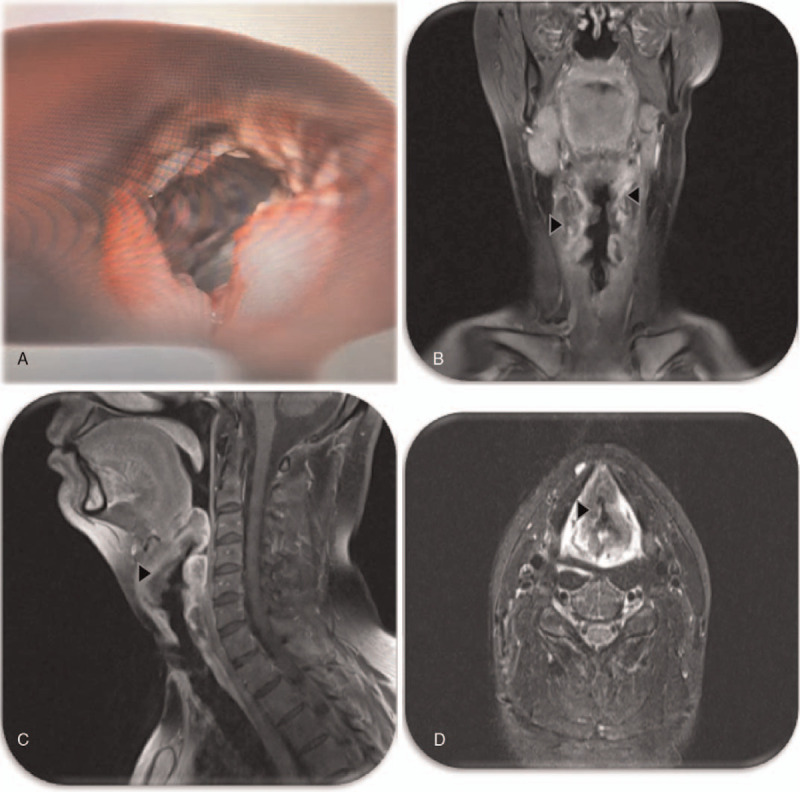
(A) Direct laryngoscopy examination with ulcerative and necrotic lesions at the epiglottis and larynx and loss of the larynx's structure. (B-D) MRI of the neck with an inflammatory compromise of the glottis and epiglottis partially obliterating the airway (arrow head).
